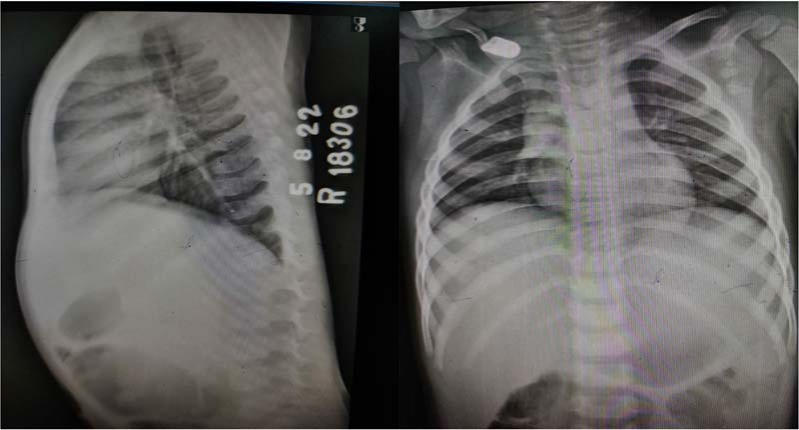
X-ray dorsal spine anteroposterior and lateral views, showing no significant abnormality.
